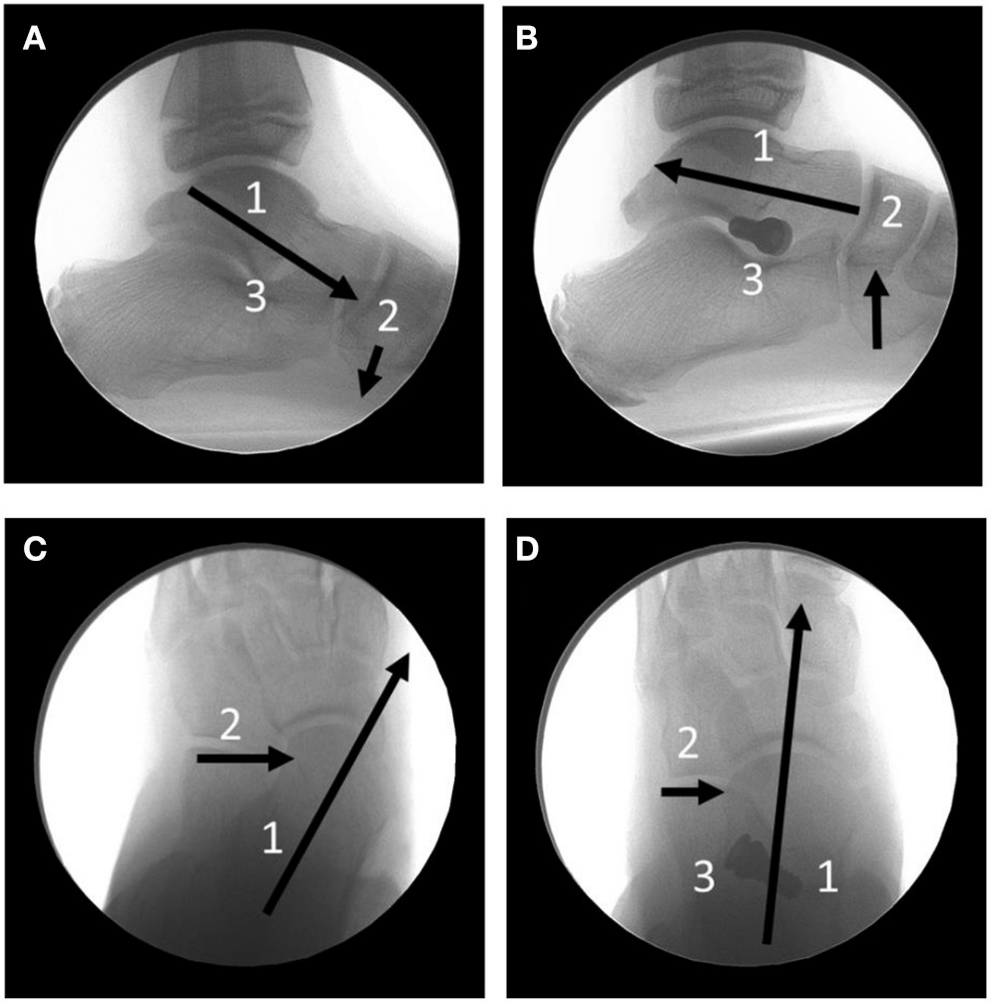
Full Weightbearing, relaxed stance position fluoroscopic radiographs. (A) Lateral image showing the anterioplantar parital dislocation of the talus (1) on the calcaneus. The navicular (2) is forced plantarly. The sinus tarsi (3) is obliterated. (B) The same foot post-extraosseous talotarsal stabilization. Notice the realignment of the talus on the calcaneus (1) and elevated navicular (2). (3) is the HyProCure (GraMedica, Michigan, USA) sinus tarsi implant. (C) Dorsoplantar view of the hindfoot. The talus (1) is medially and anteriorly displaced on the calcaneus (2). (D) The same foot post-extraosseous talotarsal stabilization. Notice the lateralization of the talus (1) and reduction of the anteriomedial displacement of the talus (2). (3) The HyProCure implant.
